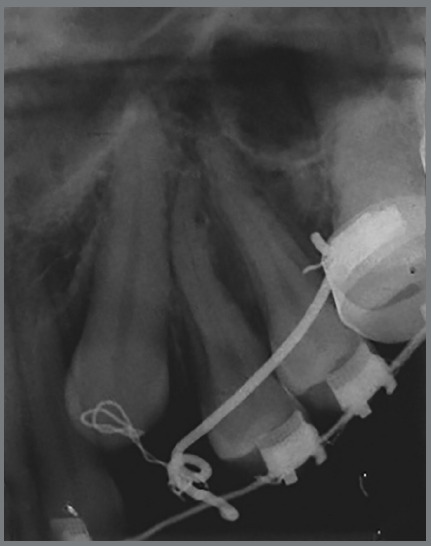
Periapical radiograph showing the line of action of the force.
